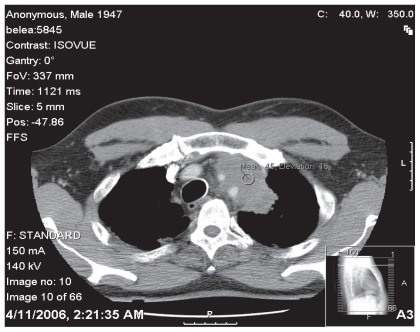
Chest CT demonstrating mass with involvement of aortic arch.
